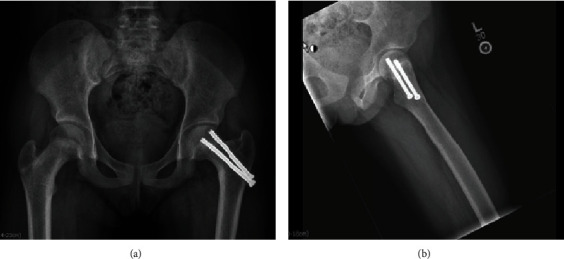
X-rays taken at 5 months postop demonstrate healing of the femoral neck fracture in some varus with some shortening of the femoral neck.
